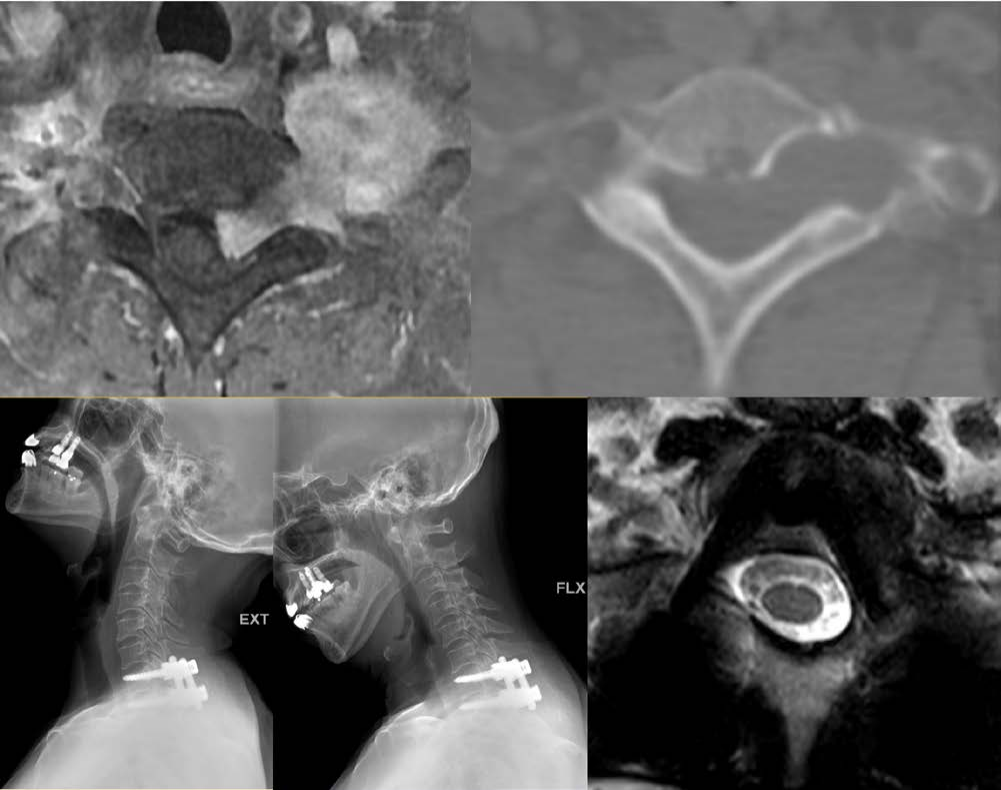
A 60-yr-old female patient with a dumbbell schwannoma on C7–T1, Lt., underwent total tumor resection and C7–T1 fusion. Postoperative 3-year dynamic X-ray imaging revealed complete fusion.
